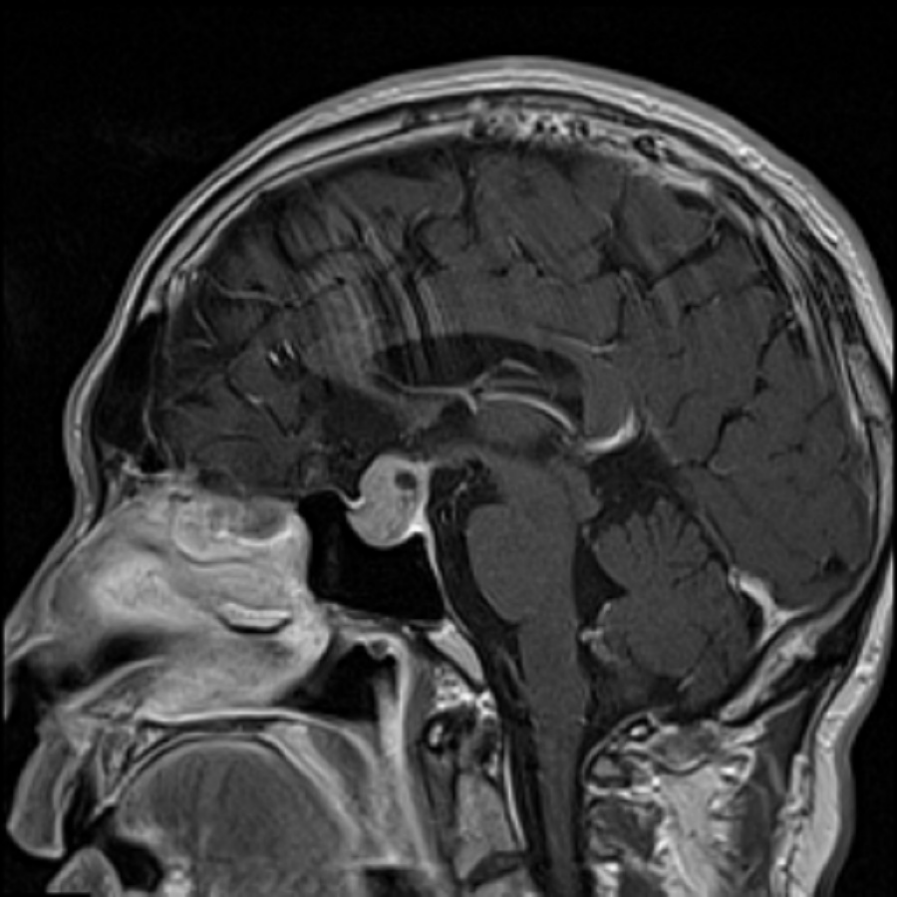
Initial MRI obtained at the time of diagnosis Initial T1 sagittal MRI again showing a sellar mass with a small cystic component in the superior aspect.
